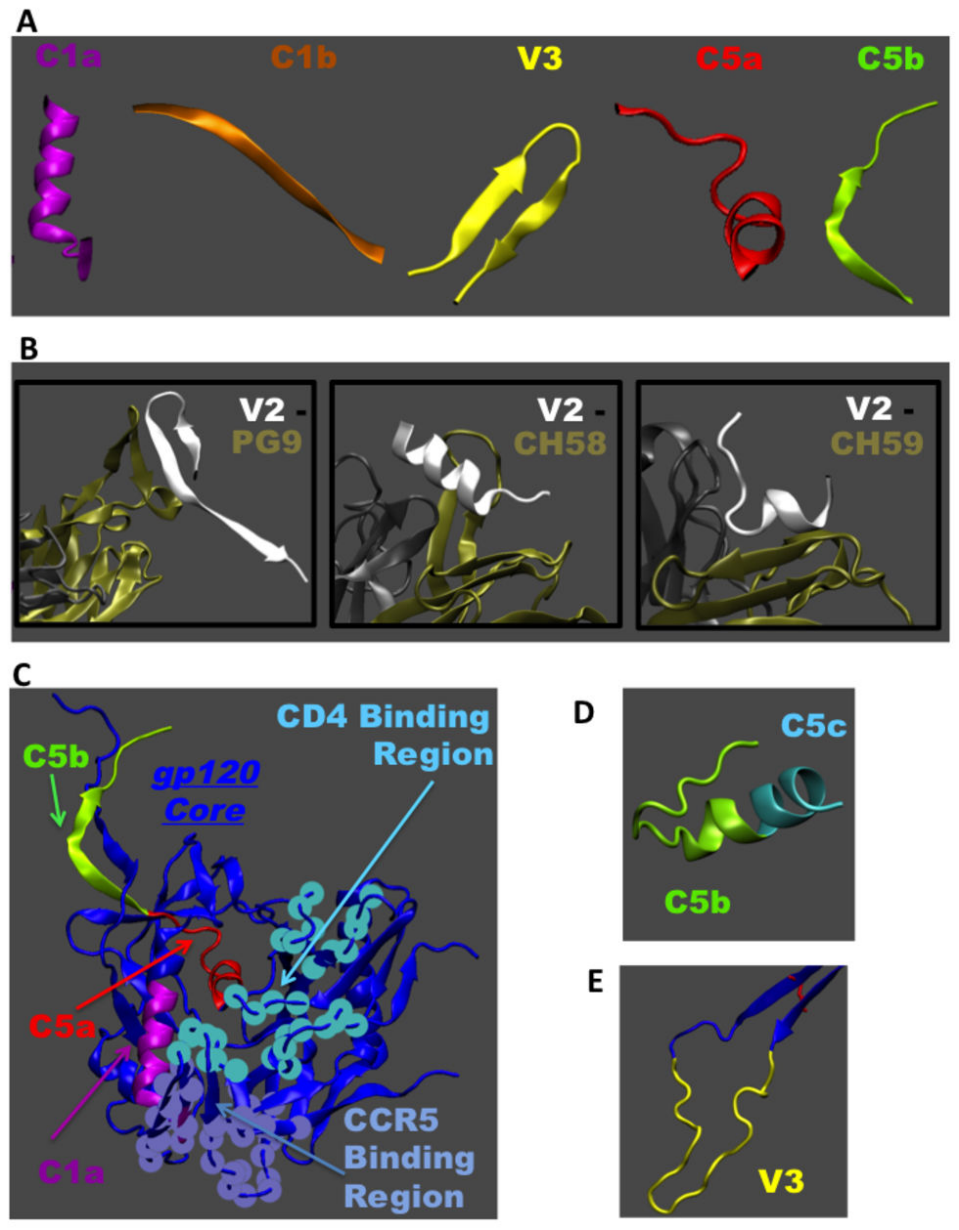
Known conformations of reactive peptides. A. Conformations of reactive peptides known from solved X-ray structures of gp120 core or fragments of gp120 in complex with antibodies. B. V2 peptide is shown with respect to different conformations it adopts depending on the bound antibody. Structures with antibodies PG9 (PDB: 3U4E), CH 58 (PDB: 4HPO) and CH 59 (PDB: 4HPY) are shown where tan and gray colors indicate the light and heavy chains of the antibody, respectively. C. The CD4 bound structure of gp120 with N-and C- terminal regions is used as a template to show the C1a, C5a, C5b peptides in the context of entire gp120 monomer structure (PDB: 3JWD). Also shown are the CD4 and CCR5 binding regions respect to these peptides. D. NMR structure of isolated region of C-terminal end of gp120 showing C5b and part of C5c peptides (PDB: 1MEQ). E. V3 peptide is shown with respect to the rest of the gp120 core (PDB: 2B4C).
